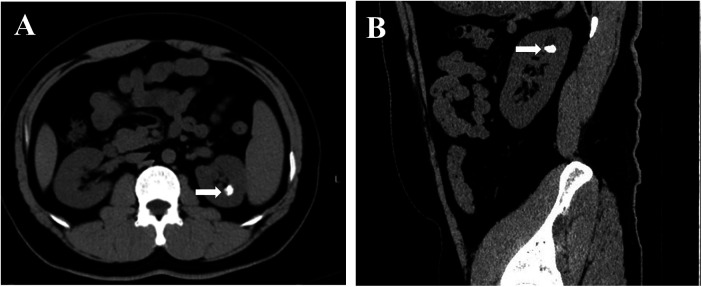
Preoperative CT imaging of calyceal diverticular calculus. (A) Coronal non-contrast CT image demonstrating a hyperdense calculus within an upper pole calyceal diverticulum of the left kidney. (B) Sagittal CT reconstruction showing the same diverticular stone in the upper-pole region.
